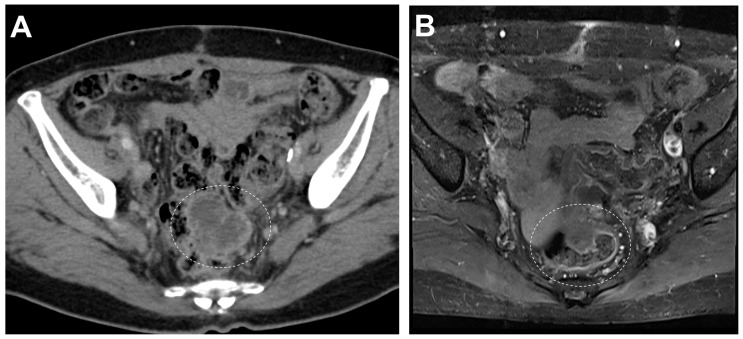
Follow-up computed tomography (A) taken four months after surgery revealed a lobulated cystic mass (dashed circle) measuring approximately 5 cm with irregular wall thickening within the pelvic cavity and papillary nodules around the vaginal stump. Pelvic MRI (B) revealed solid and cystic mass (dashed circle) and peritoneal seeding lesions up to 2 cm in diameter adjacent to the rectosigmoid junction. The treatment regimen was converted from adjuvant to palliative chemotherapy as evidence of peritoneal metastasis was found. Considering that AGCTs have been reported to have a propensity for late recurrence [29], the rapid growth of this tumor is likely attributed to a recurrence of the MLA component; furthermore, it is suggested that the collision tumor exhibits more aggressive behavior.
